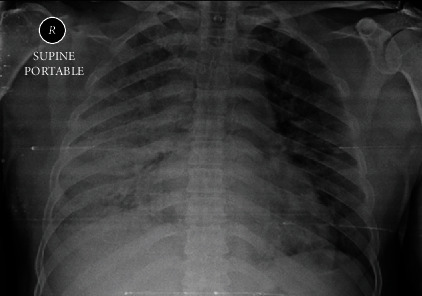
Chest X-ray of the patient from the day he was admitted to the hospital.
